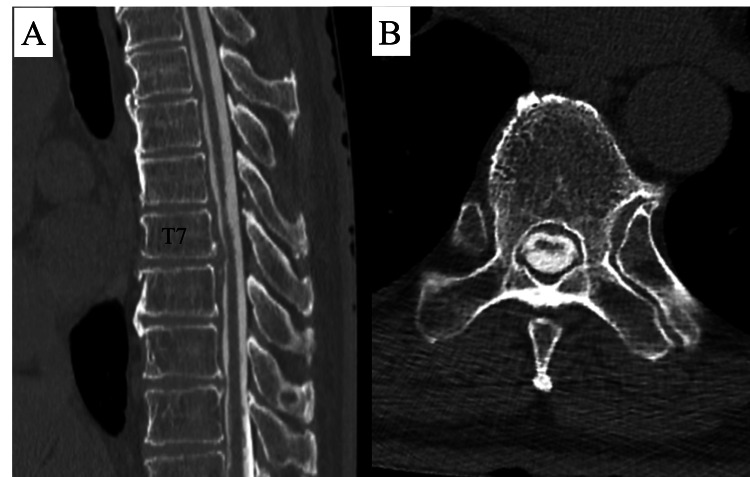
CT images of myelogram. A: Computed tomographic myelography demonstrates the atrophy and flattening of the spinal cord at T. B: A horizontal slice showed a hyperdense contrast accumulation posterior to the atrophied spinal cord.
